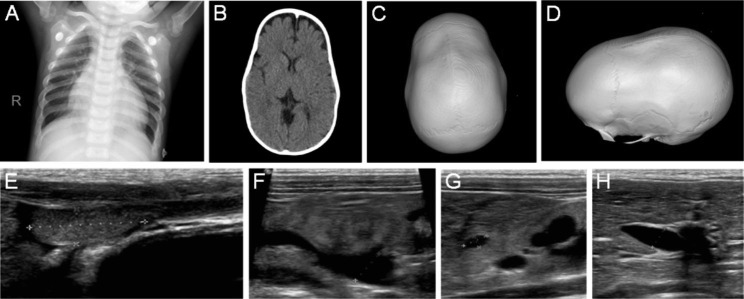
The patient at the age of 3 years and 3 months. a Frontal chest radiographs showed increased heart volume, decreased bone density, and enlarged trabeculae; b CT scan showed the bilateral frontocephalic space widened; c-d the anteroposterior diameter of the skull increased, and the sagittal suture was partially closed in three-dimensional images; e abdominal color ultrasound examination showed the right testicle located in the iliac fossa, f the liver volume was increased, the parenchymal echo was dense and enhanced, and the intrahepatic bile duct was widened, g the intrahepatic hypoechoic mass was enlarged, h enhanced kidney parenchymal echo, unclear demarcation of cortex and medulla, and hydronephrosis of the right kidney
